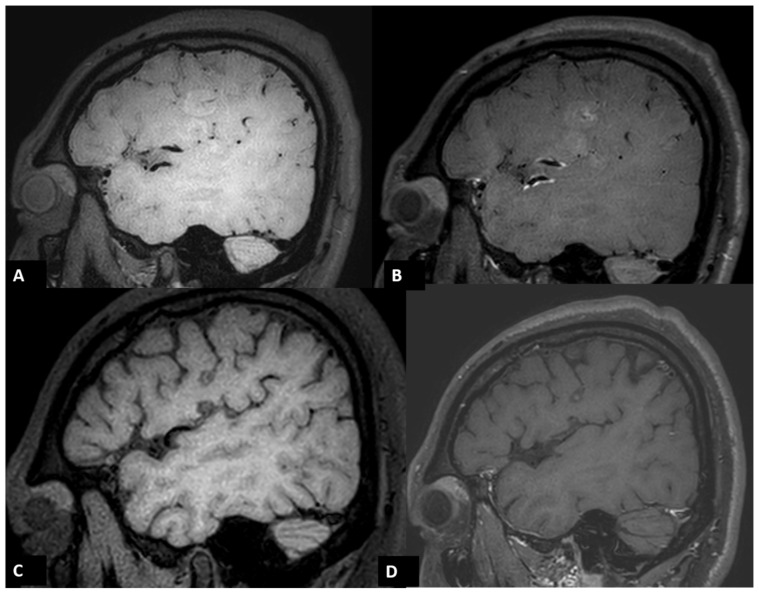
Post-treatment vessel wall enhancement in pre- and post-Gd 3D black blood T1WI images of vessel wall MRI. Concentric vessel wall enhancement of distal left MCA vessel wall (distal to the stent retriever location) (B) without abnormal signal on pre-contrast T1WI (A) due to post-EVT induced inflammatory process was seen via MRI three days after EVT. The contrast enhancement returned to normal in pre- (C) and post-Gd 3D black blood T1WI images (D) performed at the three-month follow-up.
